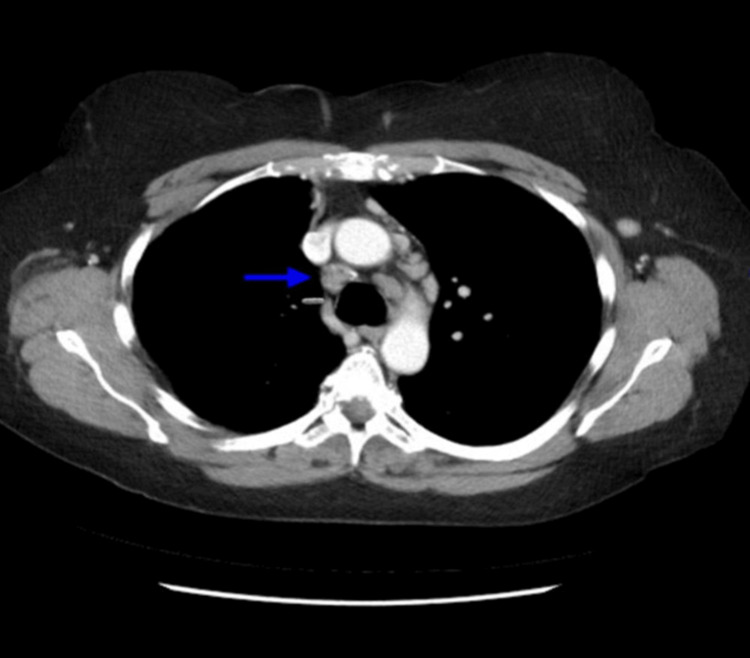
CT chest with contrast. Blue arrow showing mediastinal lymphadenopathy CT: Computed tomography
